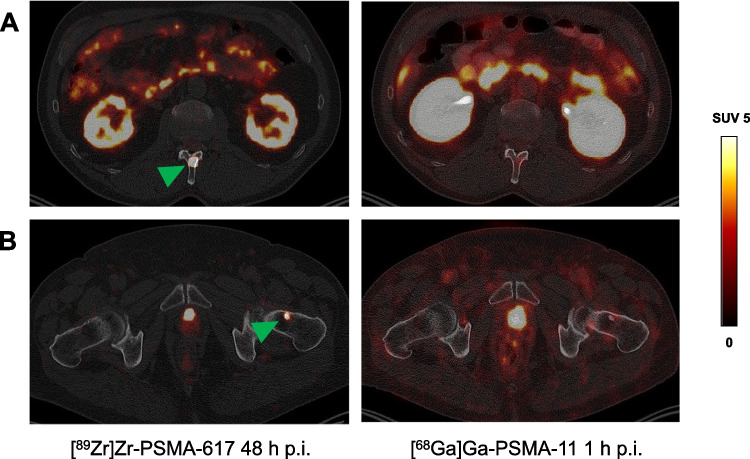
Transversal slice images showing suspected bone metastases of prostate cancer (green arrows) revealed by [^89^Zr]Zr-PSMA-617 PET/CT (left-hand column) but not discernible on [^68^Ga]Ga-PSMA-11 PET/CT (right-hand column) in two patients (rows A and B, respectively). p.i., post-injection; SUV, standardized uptake value
